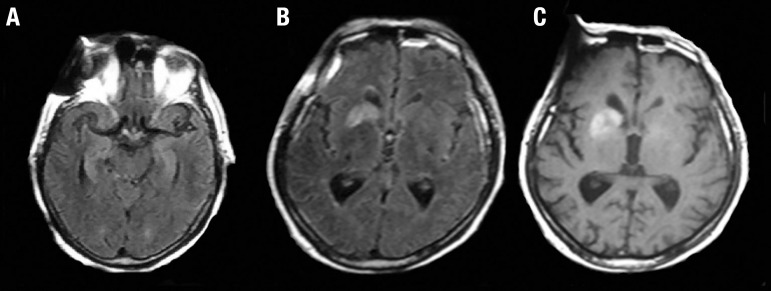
Brain MRI (case 1) showing T2/FLAIR hyperintensities in both mesial
